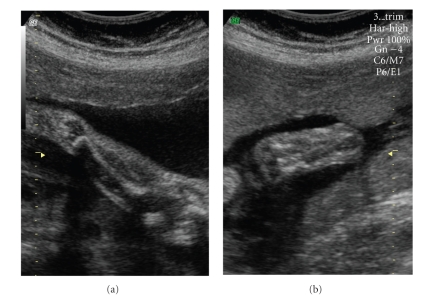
Ultrasound images showing absence of right fibula (a) and absence of the fourth and fifth right foot rays and digits (b) at 31 weeks gestation.
